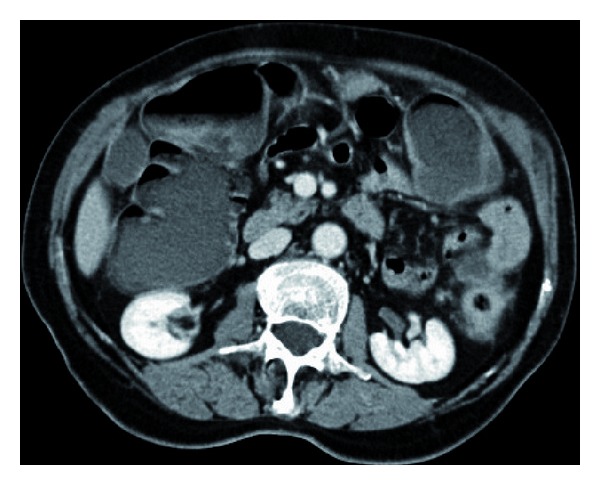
CT scan of abdomen in a patient with obstructing carcinoma of the sigmoid colon.
